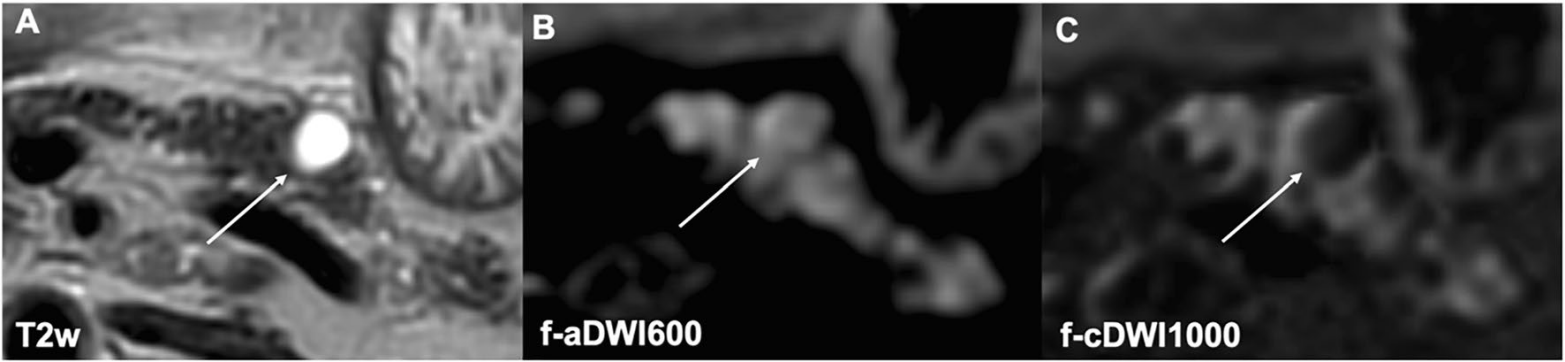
A branch-duct IPMN in the pancreatic body is depicted in the axial T2w image (A). Better fluid suppression within the lesion is seen in the high-*b*-value computed image (C) compared to the standard *b*-value DWI (B)
